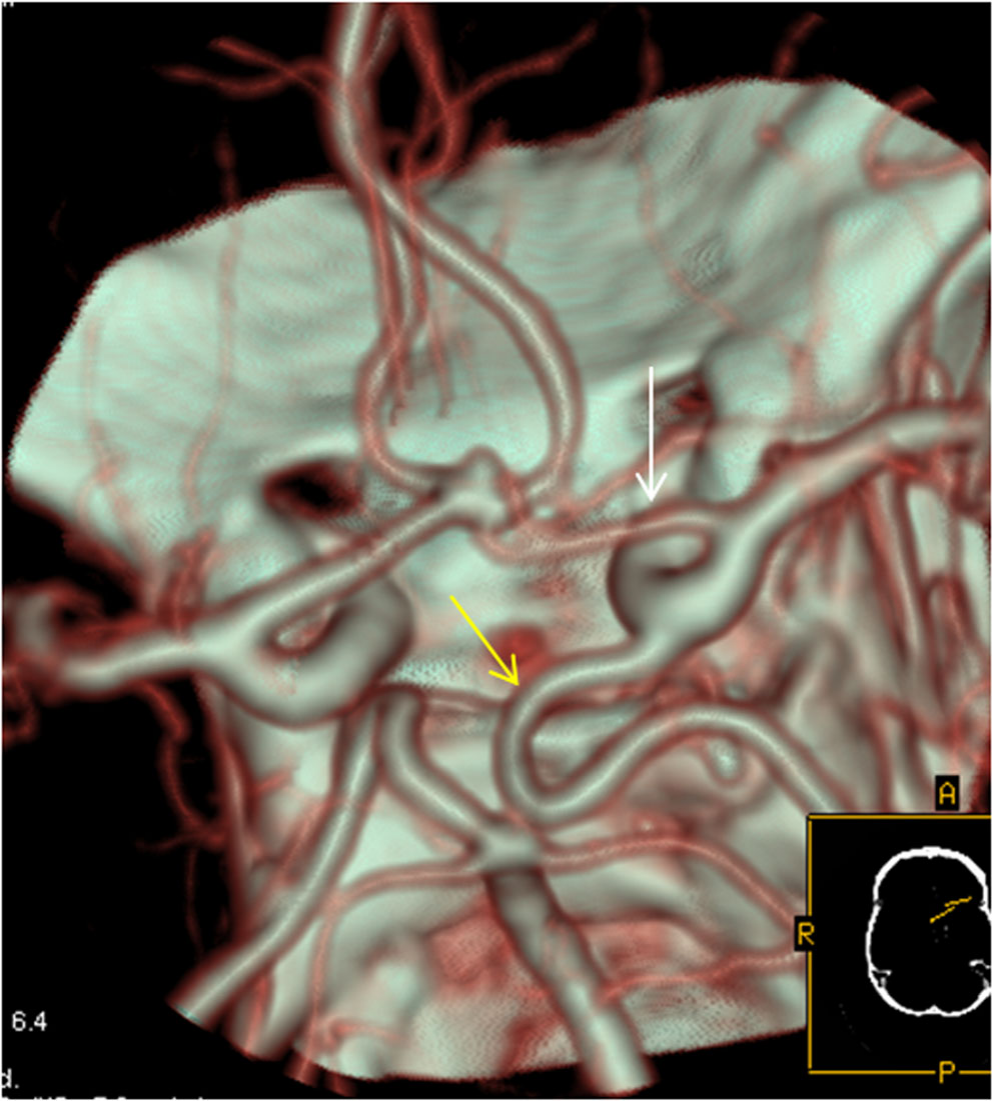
CTA (VRT 3D reconstructions) shows a hypoplastic right-sided A1 segment of the anterior cerebral artery (*white arrow*) in a patient with an Acom aneurysm. Note the presence of a foetal Pcom on the same side (*yellow arrow*)
